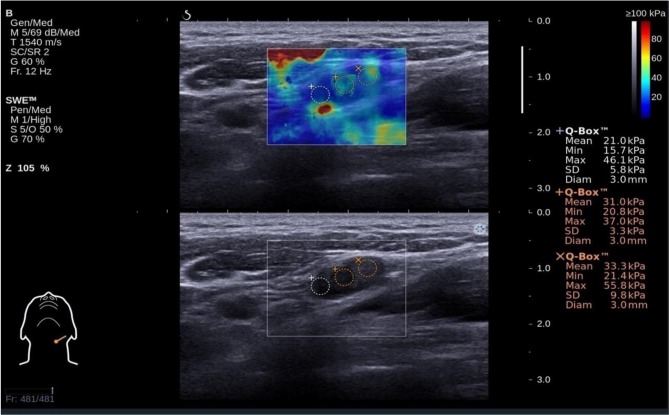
Shear wave elastography evaluation of a metastatic small cervical lymph node (sCLN) biopsy-proven: The shear wave elastography image (up) is presented simultaneously with the gray-scale ultrasound image (below) on the same screen. Shear wave elastography image revealed markedly heterogeneous color between the node lesion and surrounding tissues. The lesion was coded re-yellow. Surrounding tissues were almost pure blue in color. Emin (Minimum elasticity values) = 21.4 kPa, Emax (Maximum elasticity values) = 55.8 kPa, and Emean (Mean elasticity values) = 33.3 kPa.
